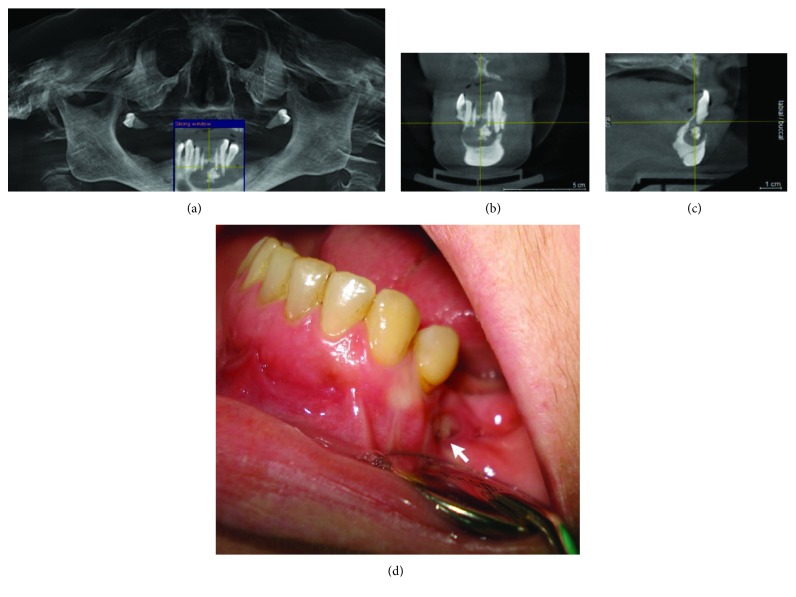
CBCT image: (a) panoramic view, (b) axial view, and (c) cross-sectional view. (d) Fenestration on the mucous membrane.
